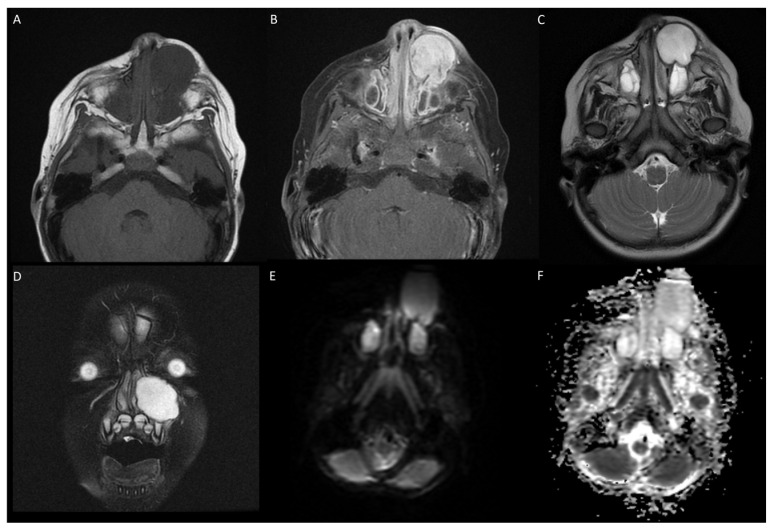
(A) T1 weighted image showing hypointense lesion in left maxillary region extending into infraorbital space. (B) T1 contrast showing avid enhancement of lesion. (C) T2 weighted axial showing homogenous hyperintense lesion. (D) T2 fat-suppressed coronal. (E,F) DWI and ADC showing no abnormal diffusion restriction.
